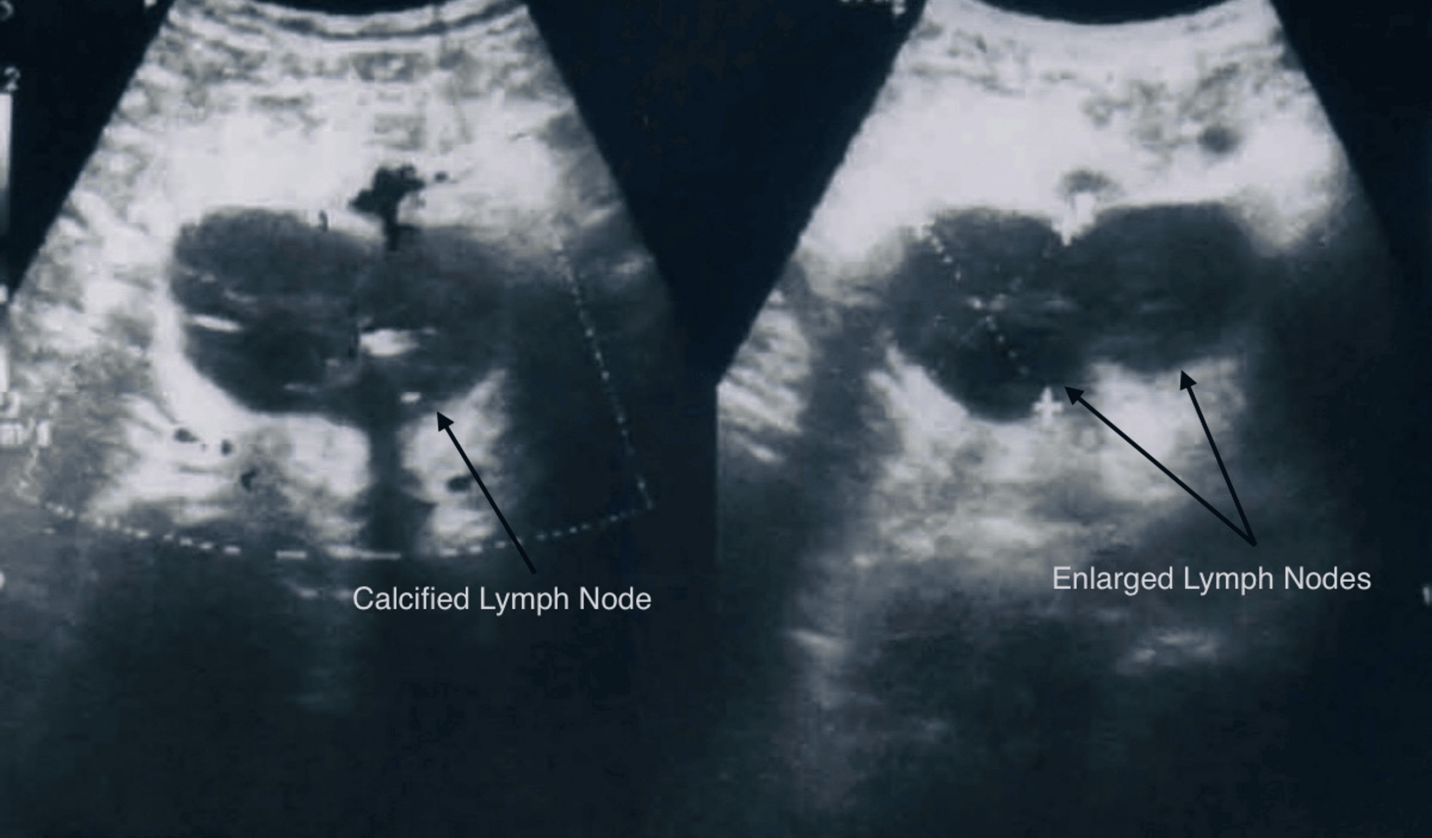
Ultrasonography Showing Lymph Node Mass
